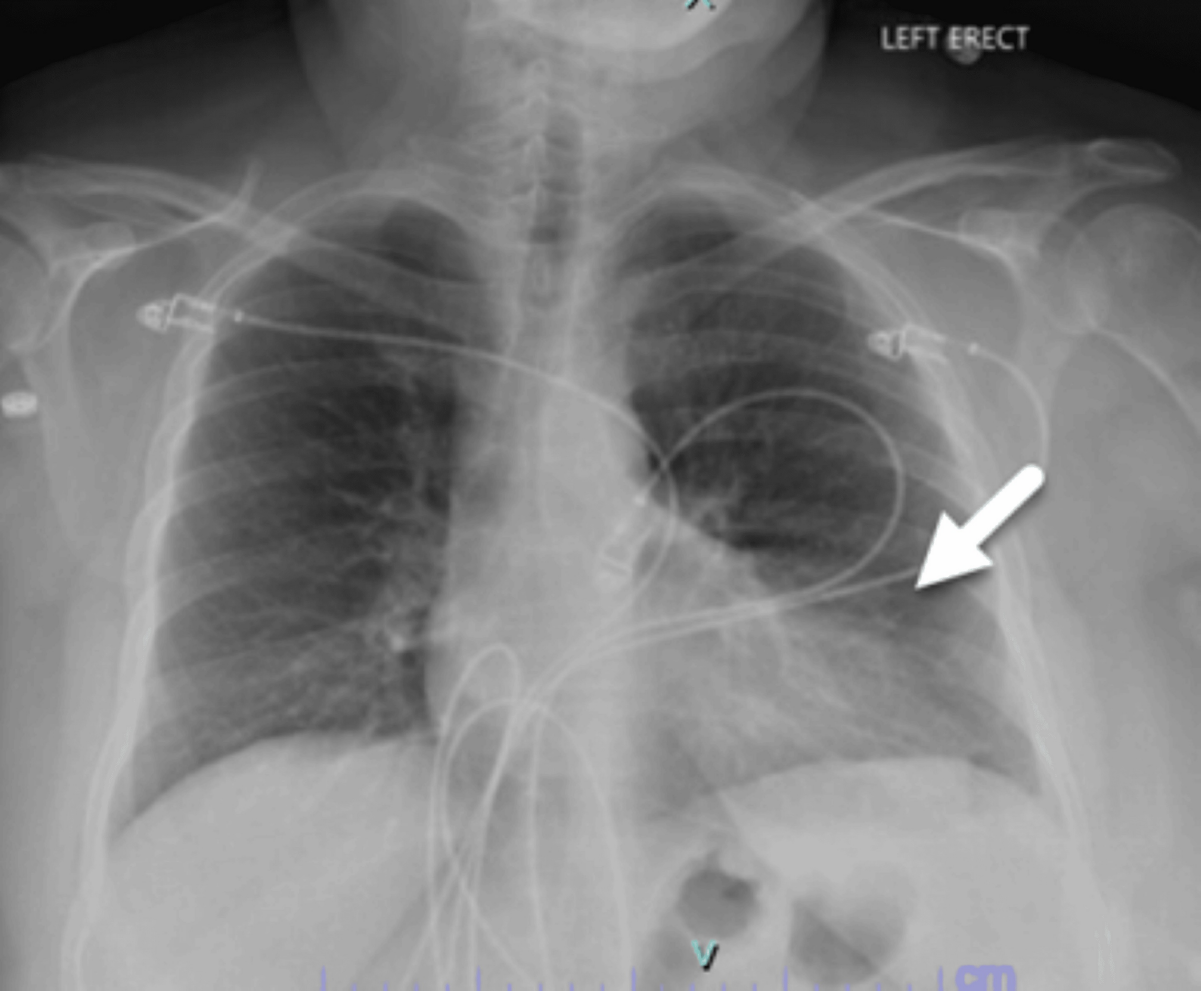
Chest radiograph showing opacity along the left cardiac border.
